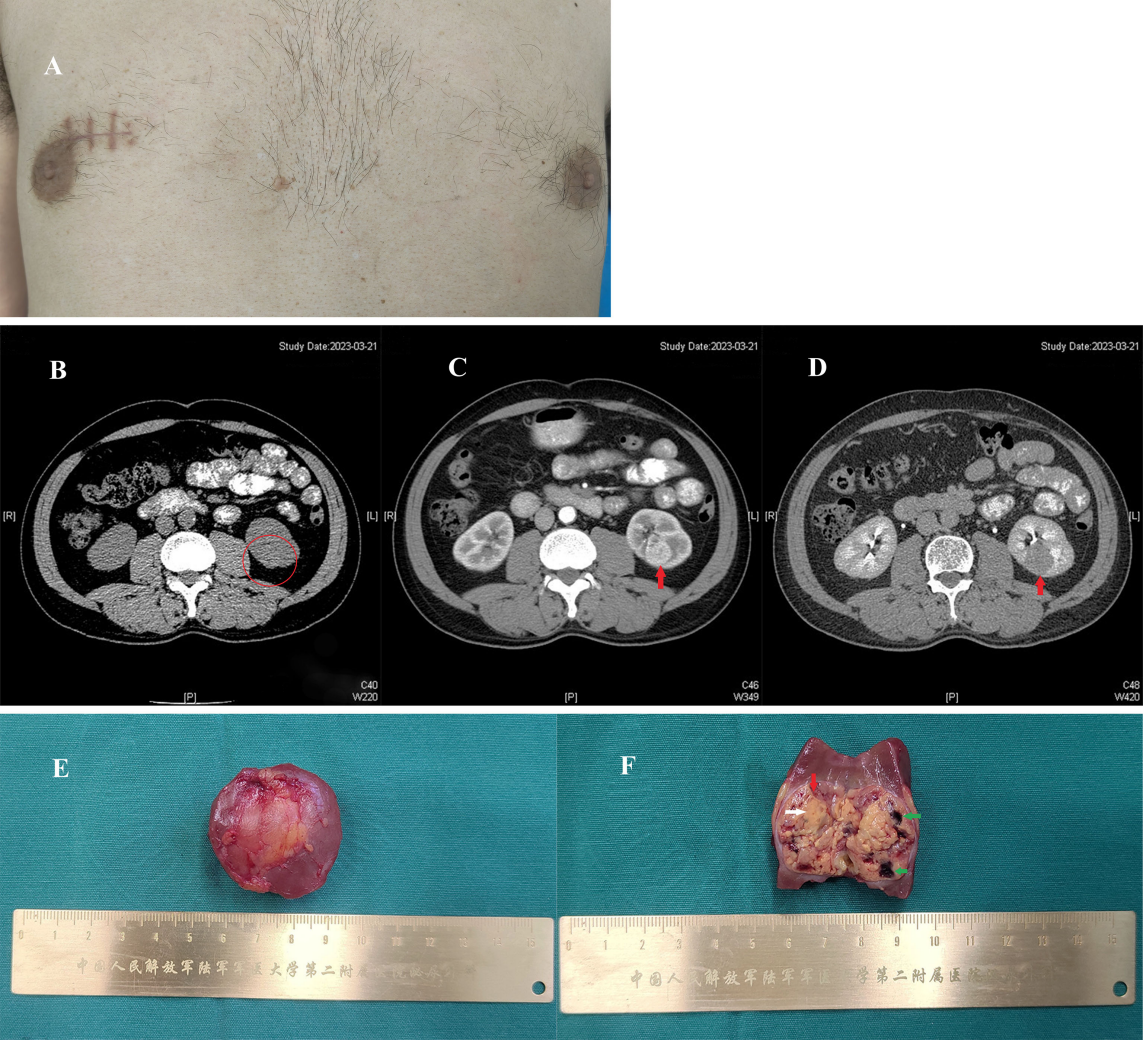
Postoperative view of the right breast after lumpectomy, dynamic contrast-enhanced CT features of the left renal mass and gross morphology of the resected left renal tumor. (A) A well-healed, approximately 2-cm linear scar is noted in the upper inner quadrant of the right breast. The surrounding skin shows normal coloration, with no palpable mass or signs of recurrence. (B) Non-contrast phase: A mass (circled in red) in the lower pole of the left kidney is iso-dense to the renal parenchyma with ill-defined margins. (C) Corticomedullary phase (enhanced): The mass (red arrow) in the posterior aspect of the lower pole shows well-defined margins and avid, heterogeneous enhancement. (D) Excretory phase: The mass (red arrow) demonstrates rapid contrast washout, becoming less dense than the persistently enhancing normal renal parenchyma. (E) Tumor tissue with partial renal tissue, measuring approximately 3 cm in diameter. (F) Sectioned surface of the tumor; the tumor is predominantly golden yellow (white arrow), with focal fibrous strands (red arrow) and hemorrhage (green arrow) observed locally.
